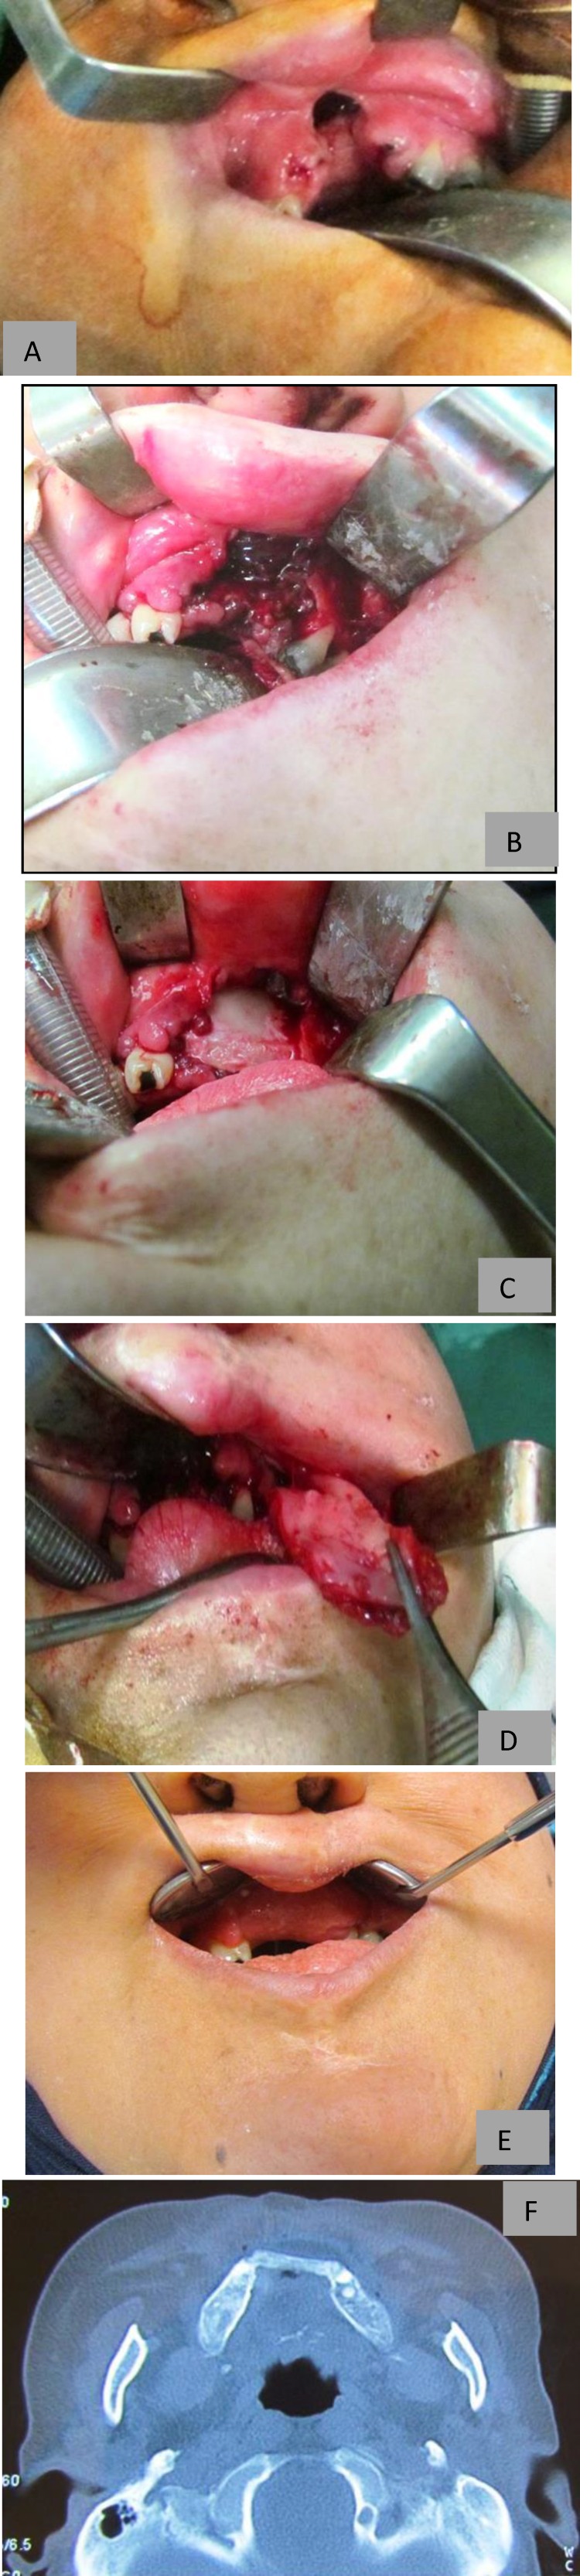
a) Permaxillectomy defect and large oronasal fistula. b) Inferior turbinate flap is used for nasal side closure. c) Bone graft wedged in between the lateral segments. d) Superiorly based flap is used for bone graft coverage. e) Photograph taken three month after operation. f) CBCT shows position of the bone graft in between lateral segments
